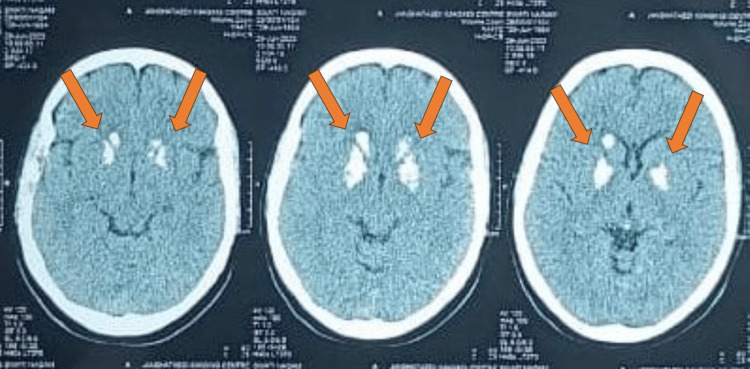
A non-contrast CT Scan of the head The arrows depict calcification in the basal ganglia.
